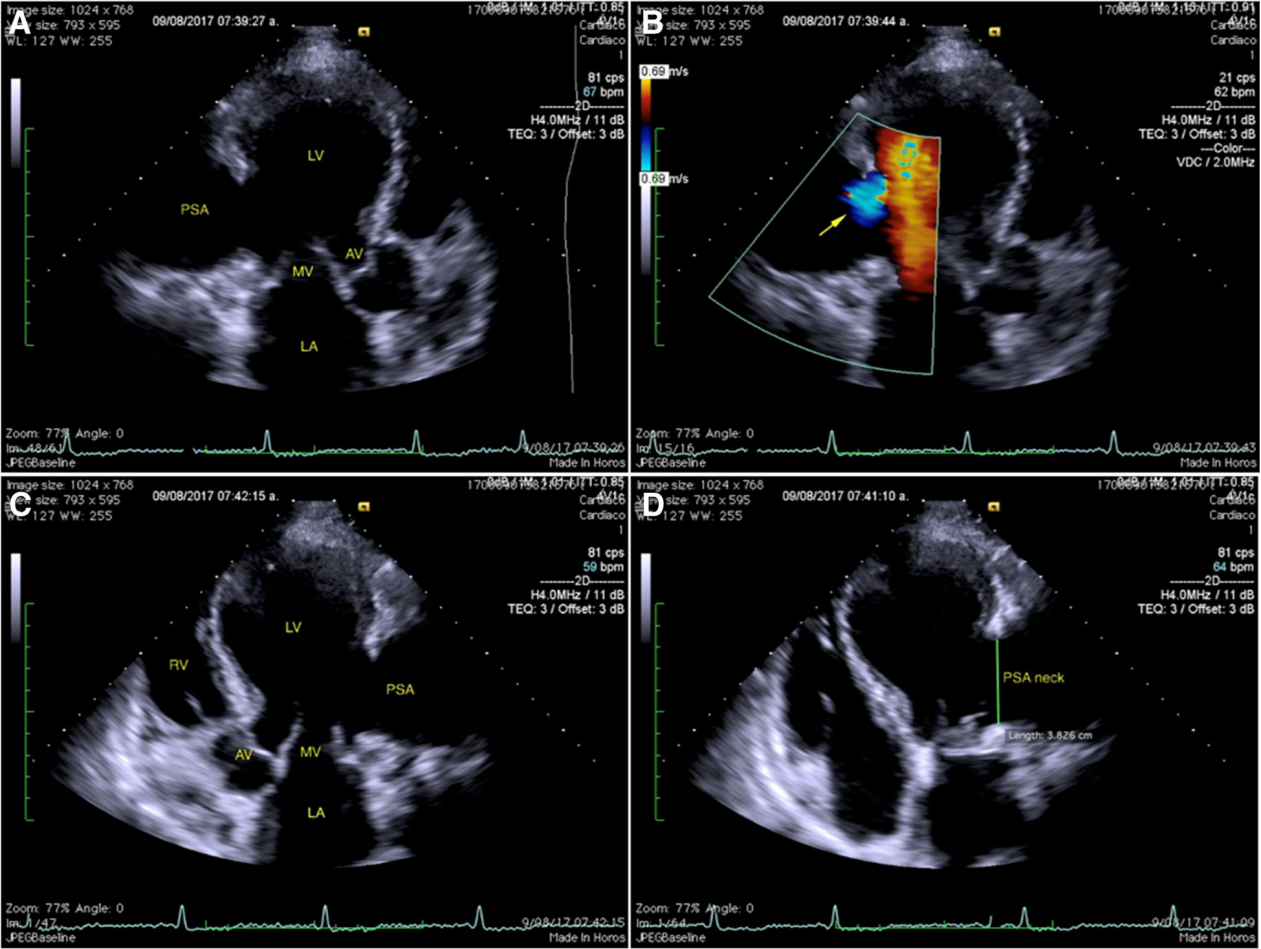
2D transthoracic echocardiogram: a Apical 3-chamber view, showing a spherical-shaped left ventricular cavity and a PSA involving the basal and mid segments of the inferolateral wall. b Apical 3-chamber view with colour Doppler showing a shunt from the LV to PSA (arrow). c Apical 5-chamber view showing a PSA involving the basal and mid segments of the anterolateral wall. d Apical 4-chamber view showing a PSA involving the basal and mid segments of the anterolateral wall with a narrow neck (38 mm)
